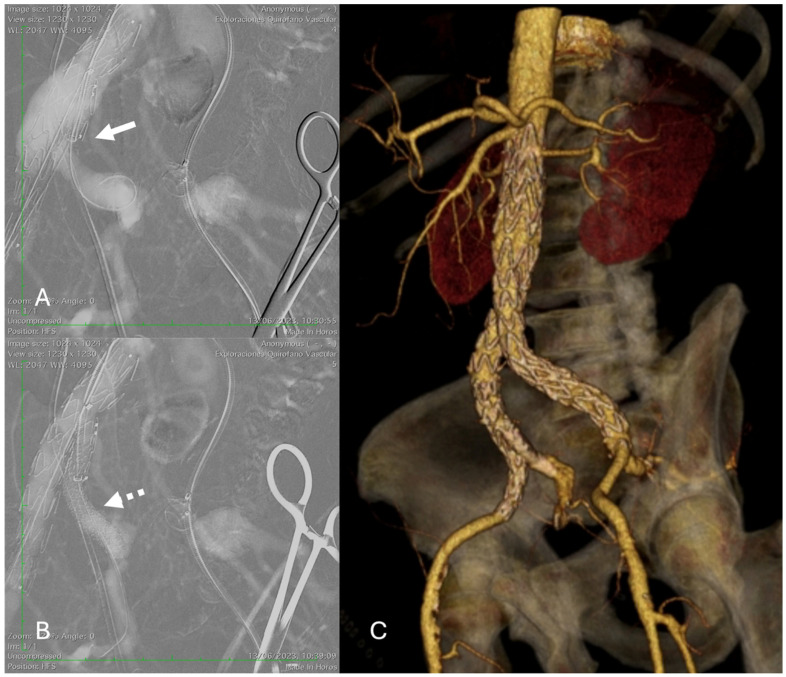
E-tegra (Artivion/Jotec) and E-liac stent-graft system (Artivion/Jotec) implantation in a patient with an infrarenal AAA and right common iliac artery aneurysm. (A) Digital subtraction CO_2_ angiography shows the main limb of the device deployed with the markers facing the iliac stump (white arrow) and cannulation of the stump and the right internal iliac artery. (B) The bridging stent (E-ventus 9 × 57 mm, Artivion/Jotec) was placed into the right internal iliac artery (dotted white arrow). (C) At 12-month follow-up, 3D reconstruction CT angiography shows successful exclusion of the aneurysms.
